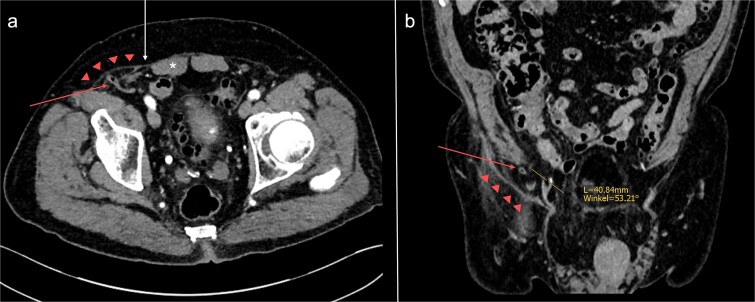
Abdominal CT-scan conducted 2 years earlier on the same patient, axial (a) and coronal (b) slices. The appendix (red arrow) is passing directly through the transversus abdominis and internal oblique muscles into the hernia sac (red triangles). The orifice is located ⁓4 cm superolateral to the right internal inguinal orifice (yellow line). *: rectus abdominis muscle; white arrow: Spigelian aponeurosis.
